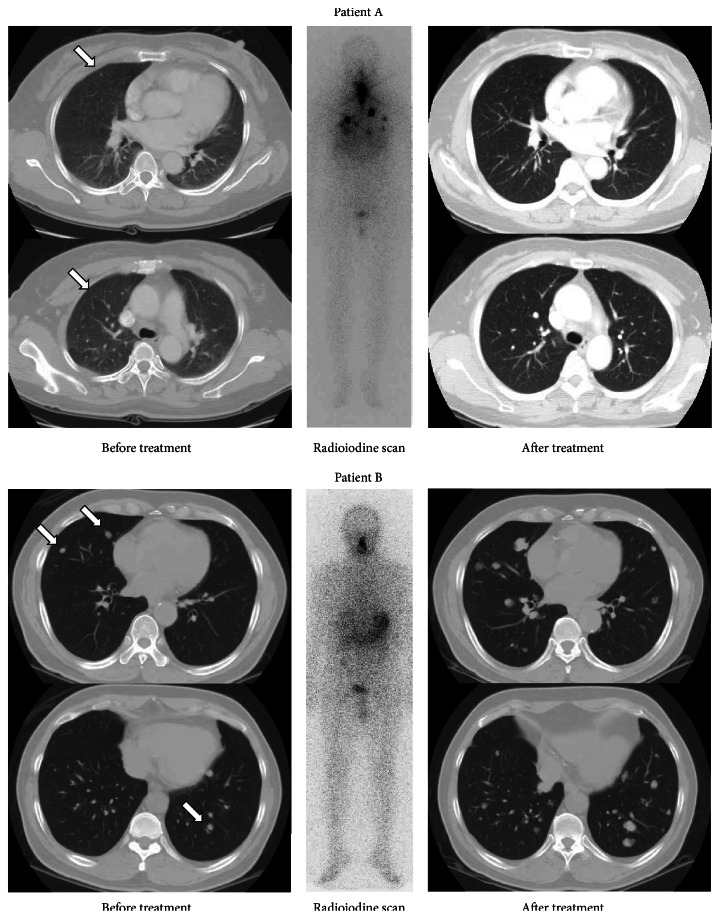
Patients A and B were diagnosed with thyroid cancer with pulmonary metastases (indicated by arrows) by pretreatment diagnostic CT imaging. Radioiodine imaging was able to forecast the therapeutic response to I-131 treatment. Patient A showed obvious uptake on radioiodine imaging and achieved complete remission after three I-131 treatments; however, patient B showed no radioiodine uptake on the imaging and progressed to disease status after I-131 treatment.
